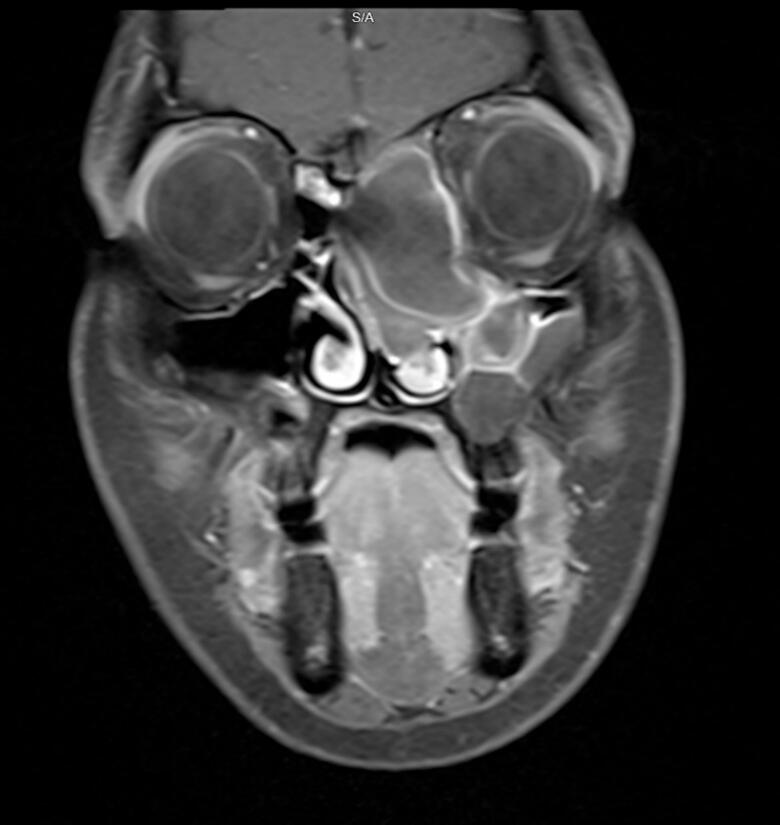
Coronal view T1 with contrast Magnetic Resonance Imaging (MRI) of paranasal sinuses showing expansion of the left nasal cavity and ethmoid the air cells with large cystic and solid component mass.
